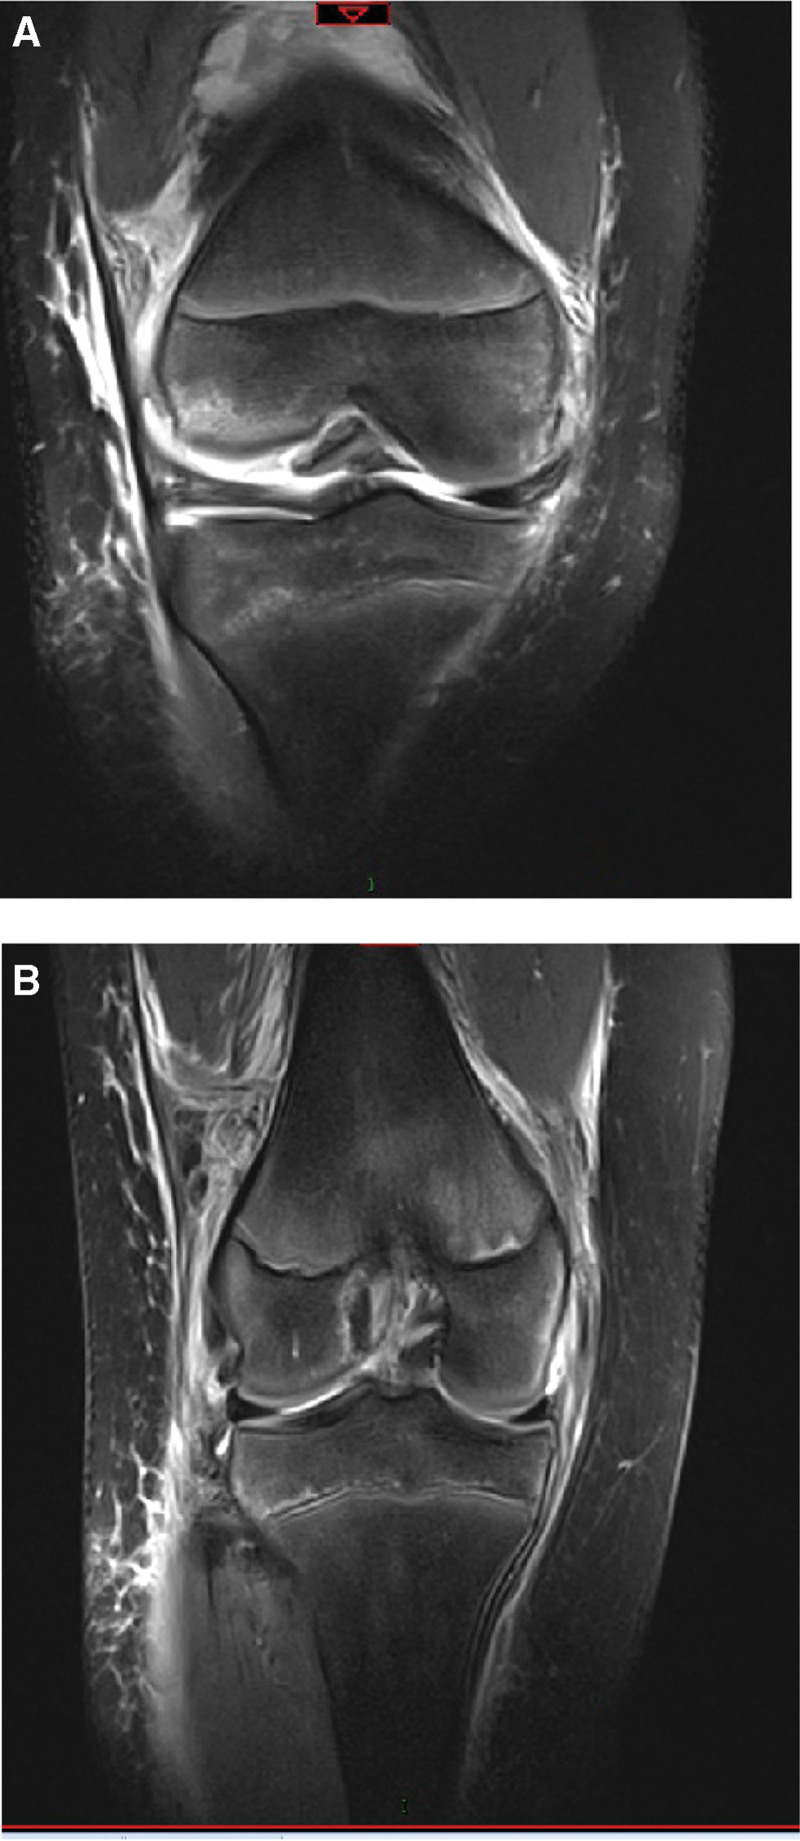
(A, B) MRI scan image of the right knee demonstrated a large lateral femoral condyle contusion with an ACL rupture near its femoral origin.
